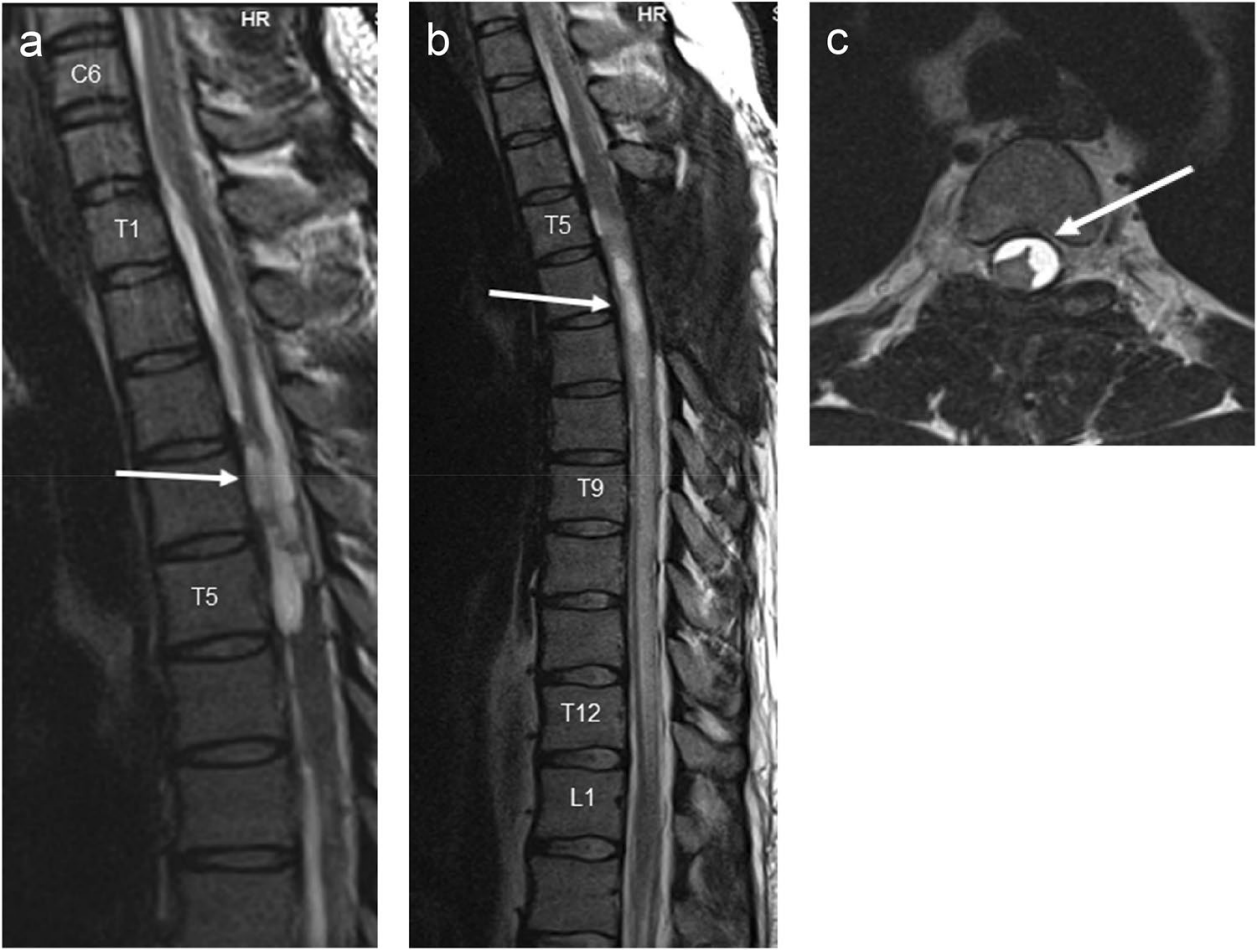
a MRI T2-weighted STIR-sagittal view of spine; intrathecal cystic mass at the T1–T5 level with contrast enhancement visible in spinal nerve roots (May 2016), b MRI T2-weighted STIR-sagittal view of spine*,*
